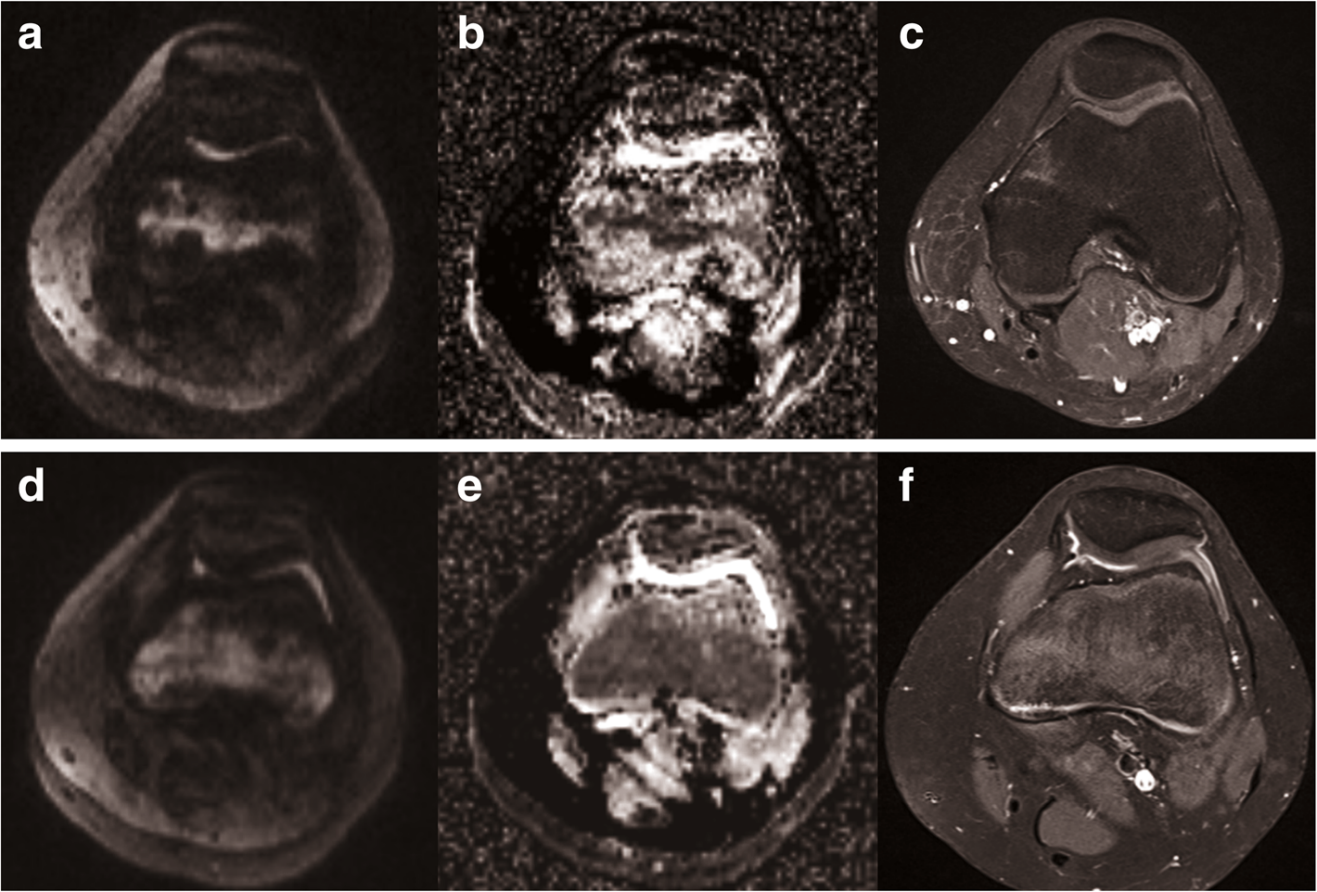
Diagnostic dilemma in a 14-year-old female control patient without synovitis (a-c) and in a 13-year-old female patient (d-f) newly diagnosed with JIA based on International League of Associations for Rheumatology (ILAR) criteria [14]. While the first patient does not show any synovial contrast enhancement (category 1, c), there is mild synovial enhancement and some synovial fluid discernable in the JIA patient (category 2, f). Both patients, however, display retropatellar and parapatellar high signal on DWI b = 800 s/mm^2^. Some signal increase as in (a) is frequently encountered in normal knee joints, possibly due to “T2w shine-through” of small amounts of physiological joint fluid, making diagnostic differentiation of synovial signal category 1 and 2 difficult, or impossible
